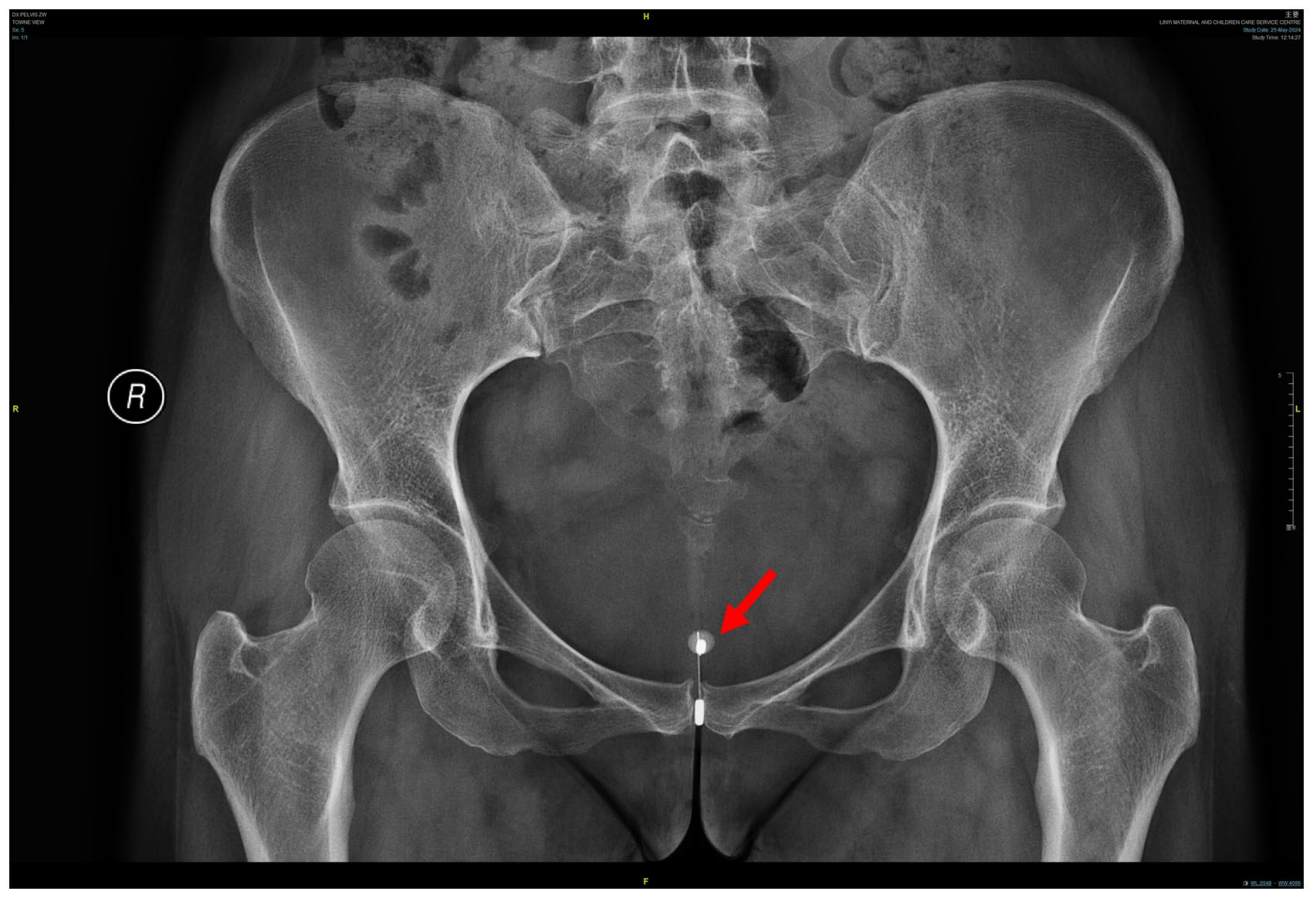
Pelvic X-ray showing the IUD, with a round, high-density shadow around one arm of the IUD, indicating bladder stone formation (red arrow). IUD, intrauterine device.
